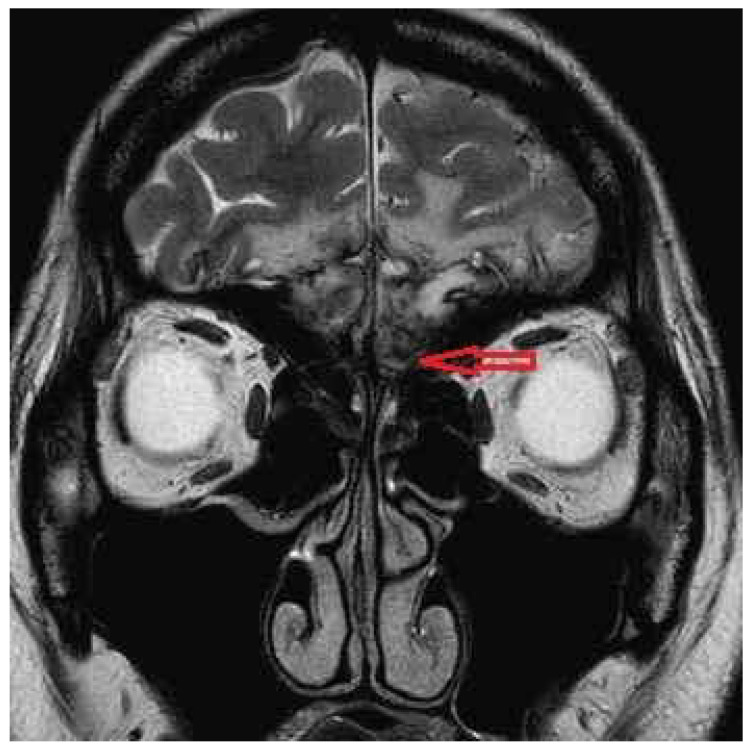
Magnetic resonance imaging showed injury to the base of the frontal lobe (red arrow).
